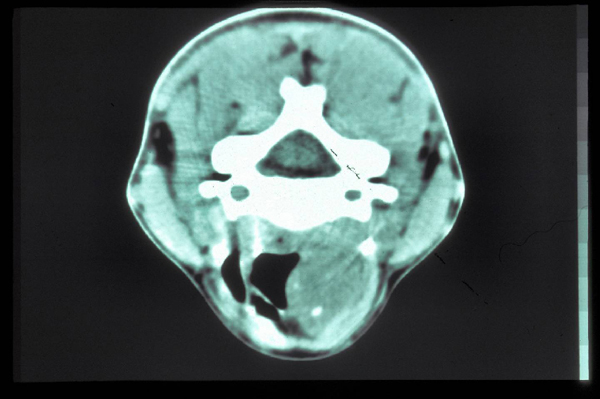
Computed tomography scan at the level of the supraglottis.
